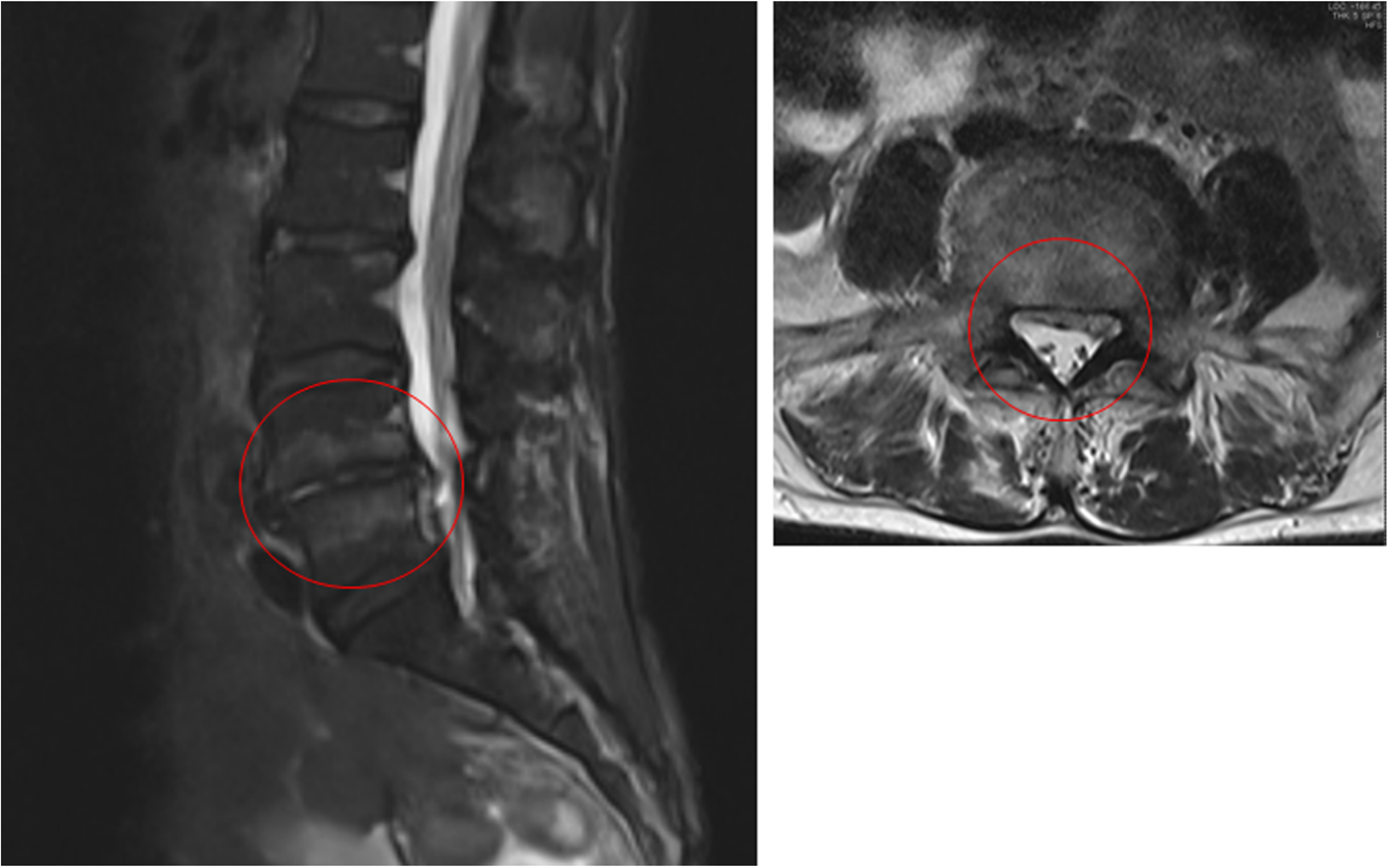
Lumbar MRI-short-tau inversion recovery (STIR) image (hospital day 3). An STIR image shows mildly hyperintense signal (red circle) in the vertebral body endplate of lumbar segments 4 and 5, which dips down on the posterior side of the 5th lumbar vertebra
